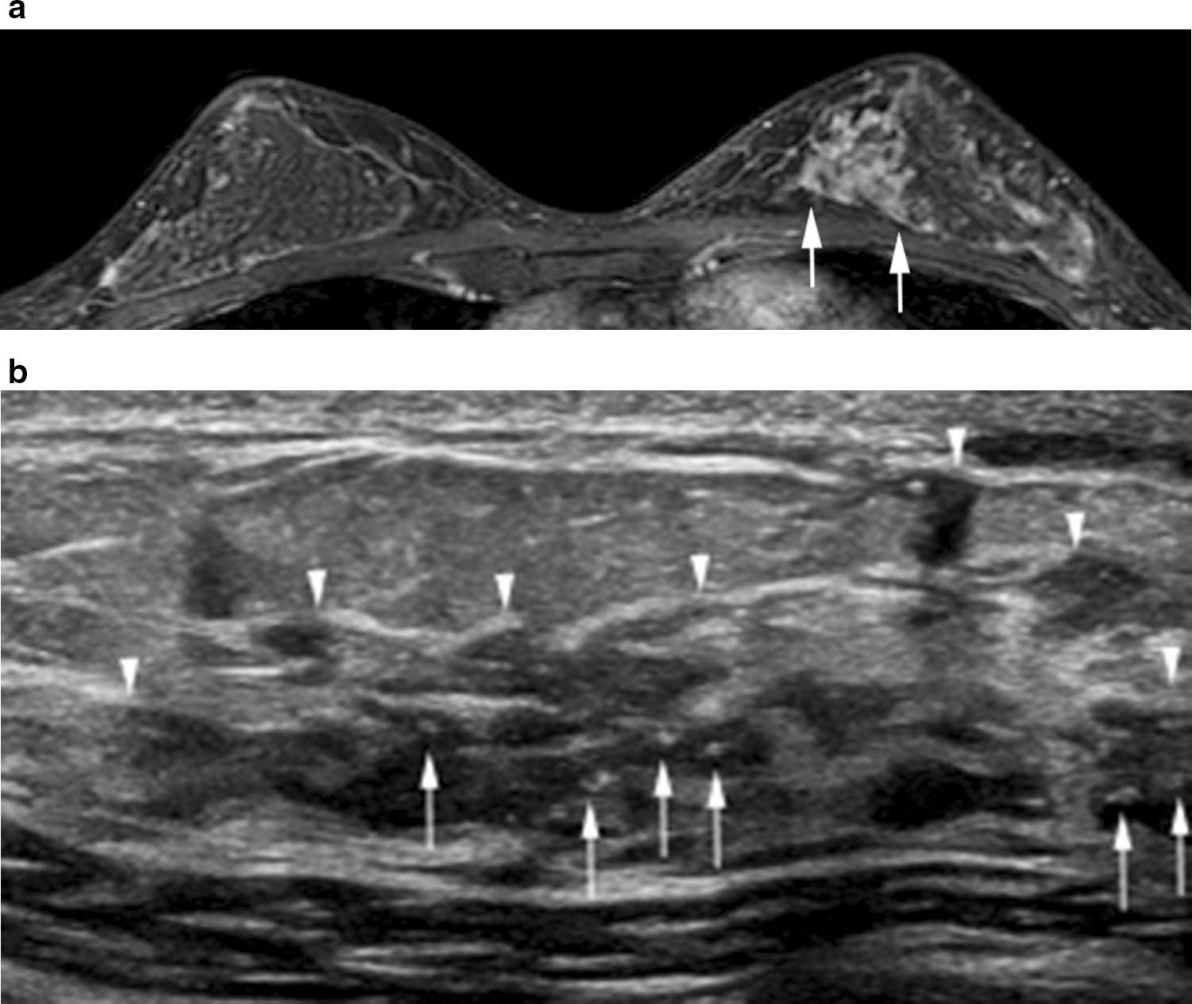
High-grade DCIS in the left upper inner breast in a 43-year-old woman. a The axial postcontrast T1-weighted image shows segmental enhancement (arrows). b The corresponding US image shows a segmental non-mass lesion (arrowheads) with associated calcifications (arrows)
